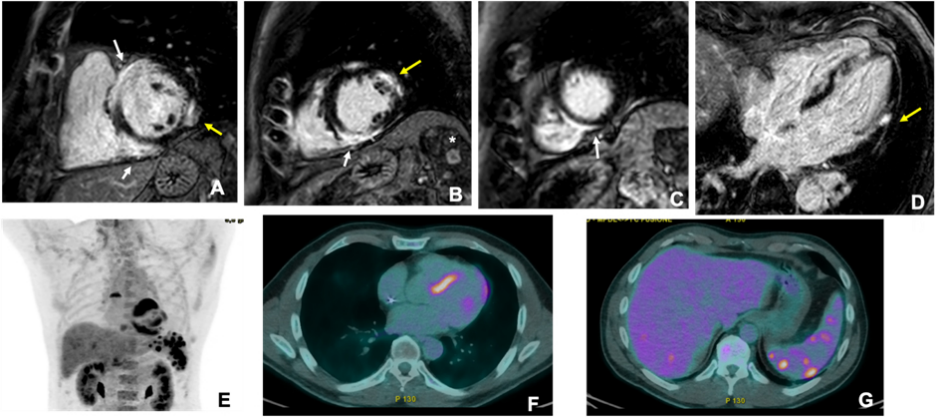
Cardiac magnetic resonance (CMR) and 18F-fludeoxyglucose (FDG)-positron emission
